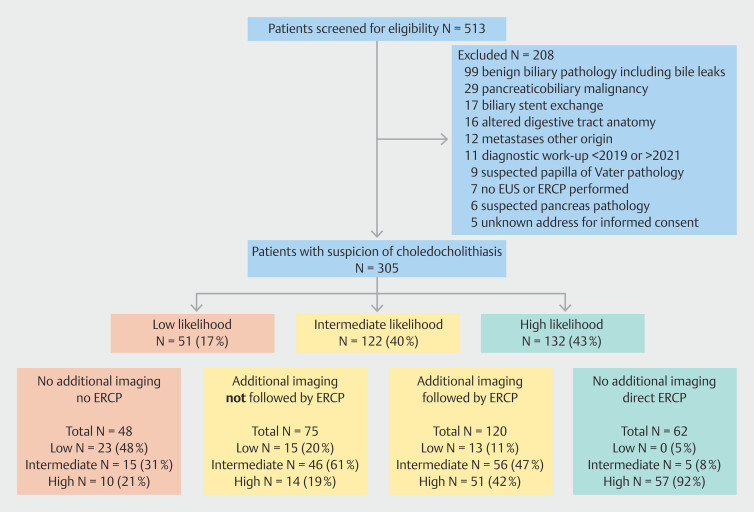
Inclusion and exclusion, likelihood groups, and treatment allocation. EUS, endoscopic ultrasound; ERCP, endoscopic retrograde cholangiopancreatography.
